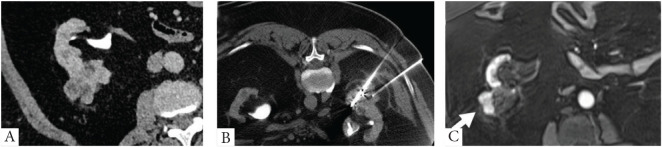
Recurrence in an Intermediate risk mRENAL score. (A) Pre-procedure contrast-enhanced CT abdomen and pelvis showing an exophytic interpolar enhanced right renal mass. (B) Intra-procedure CT showing two cryoprobes. A post-biopsy peri-renal hematoma is also noted. (C) 1.5-year post-procedural MRI follow-up shows an area of post-contrast enhancement (white arrow) concerning recurrence.
